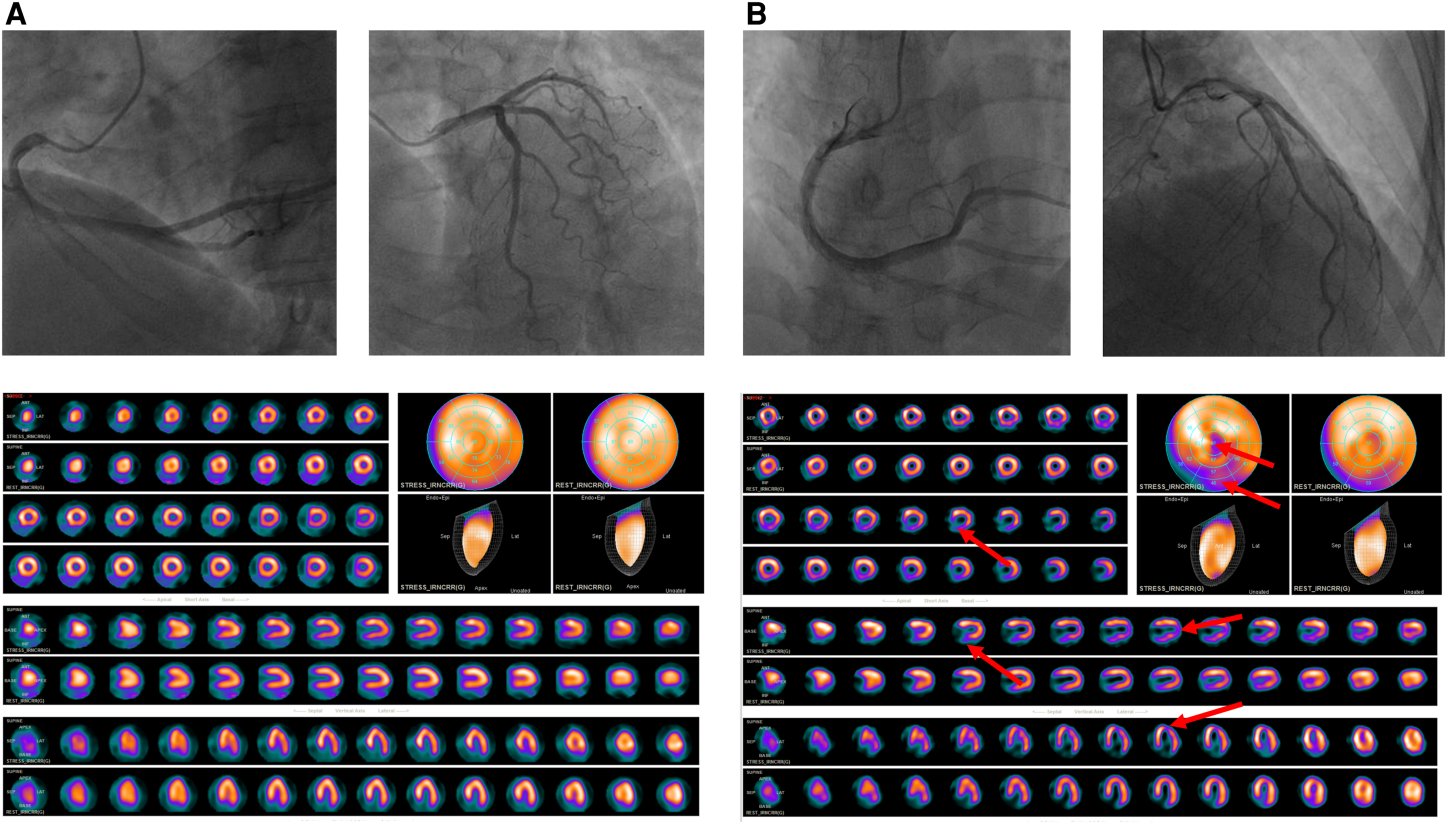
(A) A 60-year-old female patient presented to the cardiology department of our hospital for “chest pain for 2 + months”, CAG suggested that there was no stenosis in the left and right coronary arteries; SPECT MPI was normal, the SSS = 0, by the follow-up date of 25 months, the patient did not have MACE (B) A 52-year-old female patient presented to the cardiology department of our hospital for “repeated chest pain for 6 days and aggravation for 11 h”, CAG suggested that there was no stenosis in the left and right coronary arteries; SPECT MPI indicates sparse radioactive distribution of left ventricular part of the segments in stressing phase (red arrow), and filling in resting phase, considering myocardial infarction, the SSS = 9, the patient was re-hospitalized 8 months later due to angina pectoris.
